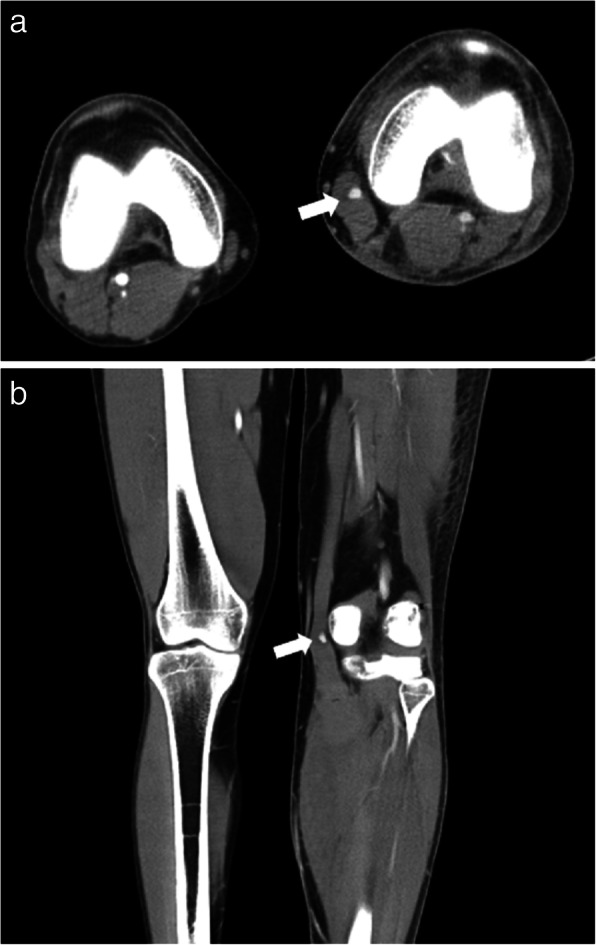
Computed tomography angiography of the lower limbs. a Axial view and b coronal view showed intramuscular hematoma and unusual focal contrast enhancement (white arrows) within the sartorius
